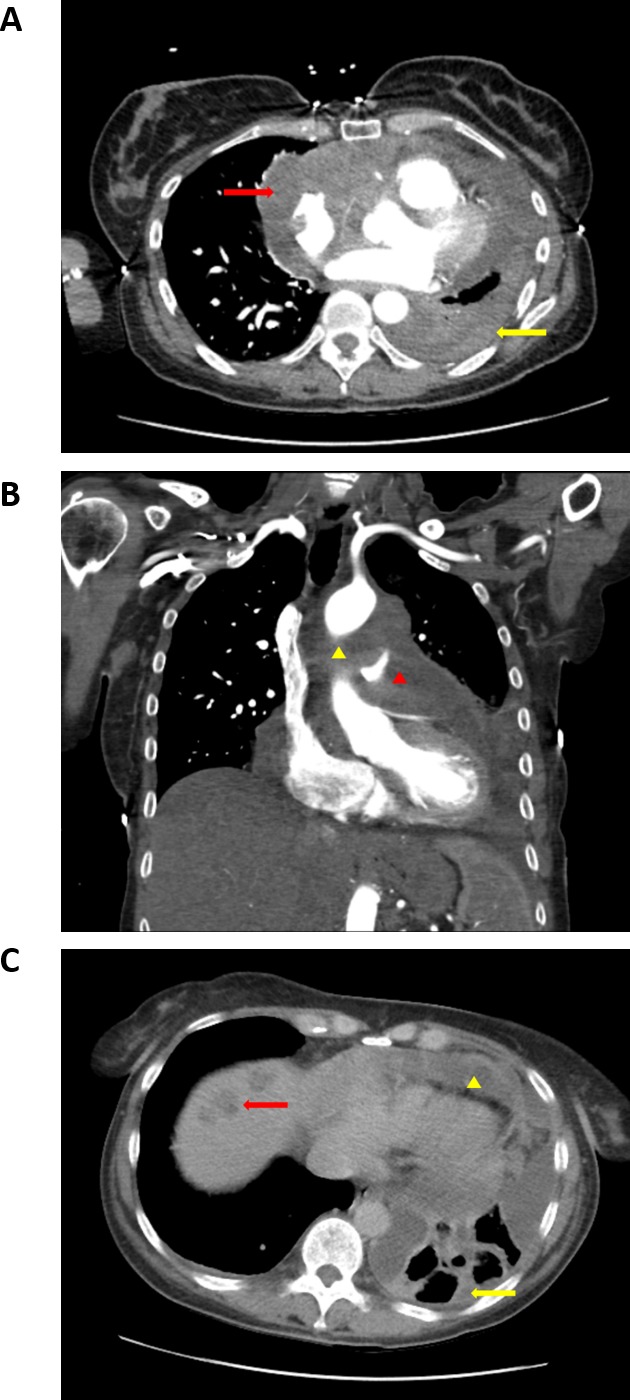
CT imaging of cardiac angiosarcoma prior to propranolol treatment (A) Baseline axial chest CT showing a large soft-tissue mass invading the right atrium and encasing the heart (red arrow). There is also nodular pleural thickening (yellow arrow) along the costal and mediastinal pleural surfaces and a small pleural effusion secondary to pleural infiltration. (B) Baseline coronal chest CT shows soft tissue mass encasing the ascending aorta (yellow arrowhead) and main pulmonary artery (yellow arrowhead). (C) Baseline axial chest CT shows circumferential pericardial thickening and effusion (yellow arrowhead) and nodular pleural thickening (yellow arrow) with loculated pleural effusion. Note small hypodense lesions in the right lobe of the liver consistent with metastases (red arrow).
